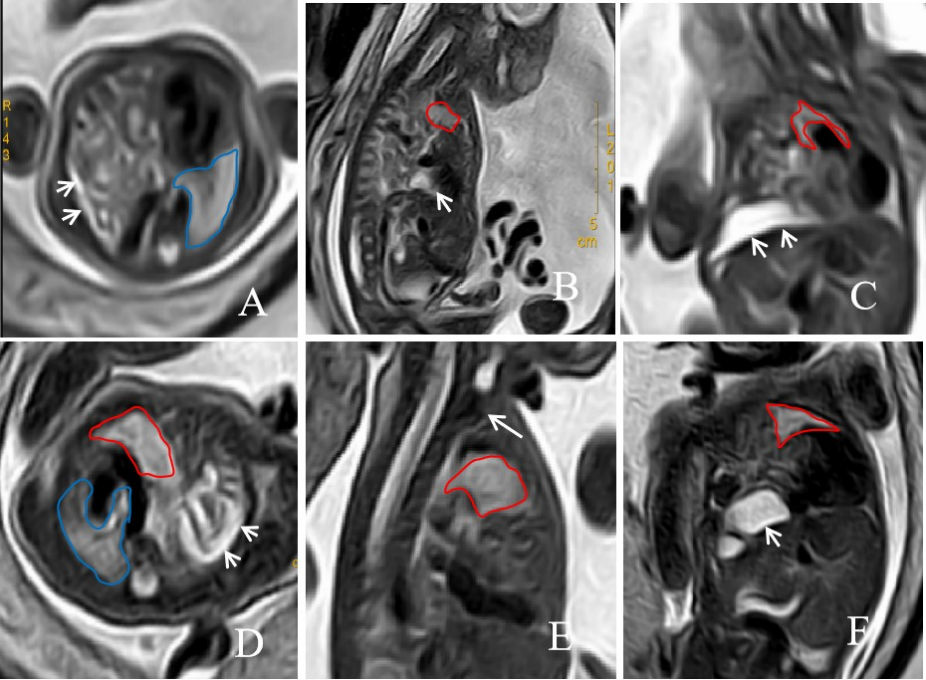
A 43-year-old pregnant woman, the fetus with left CHD on prenatal MRI. The axial, sagittal, and coronal T2-weighted images at 28w5d of gestation(A, B, and C) before FETO treatment, and at 33w0d of gestation after FETO treatment(D, E, and F) showed abdominal organs, such as the stomach and intestines, herniated into the chest cavity(short arrow). A T2-weighted image on a sagittal plane showed that (E, long arrow). The area delineated by the red line represents the left lung that has herniated into the chest cavity, while the area outlined by the blue line is the normal right lung.
